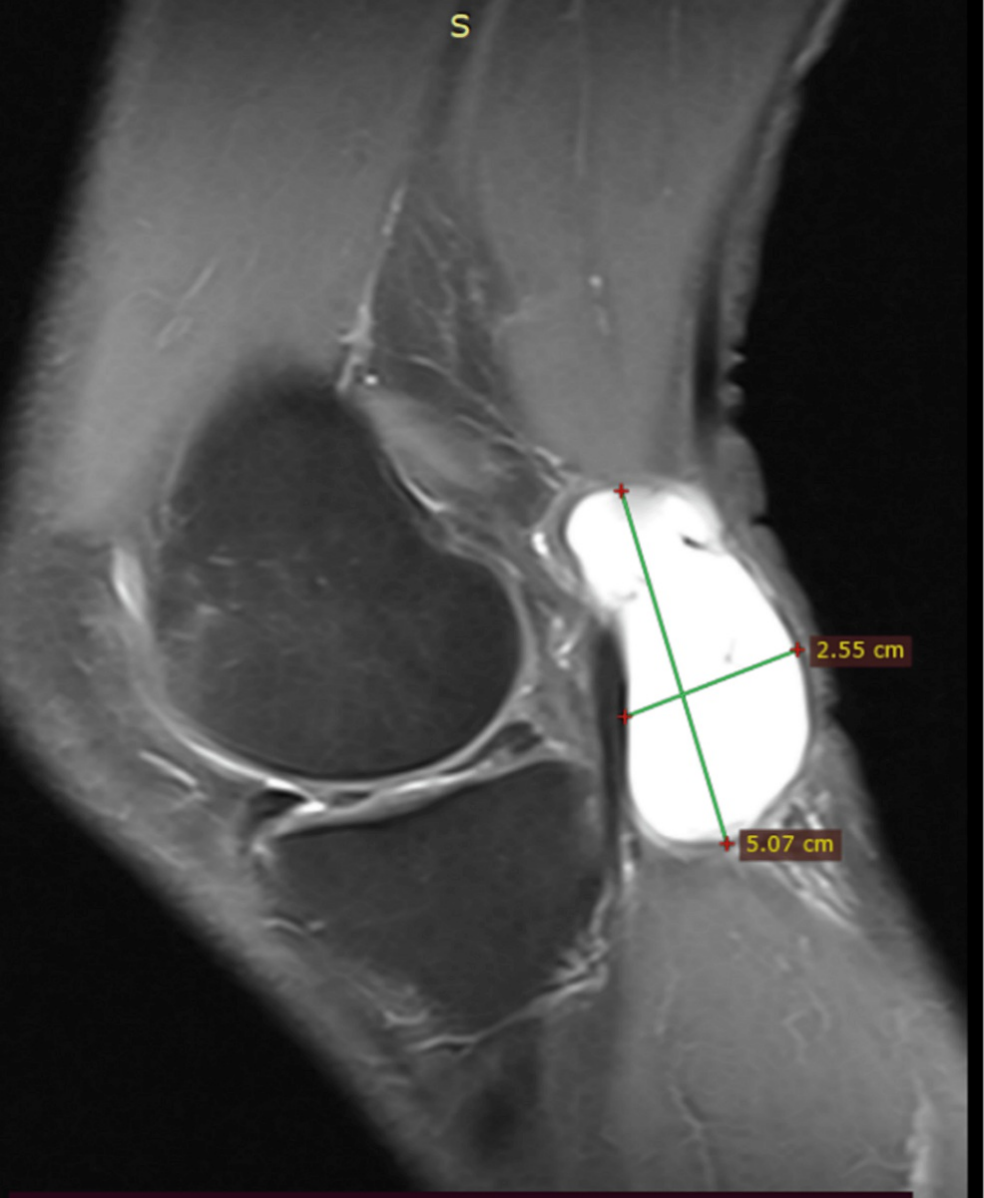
Sagittal T2-weighted image with increased signal of the cyst lying just lateral to the semimembranosus tendon in the popliteal fossa. Selected sample MRI studies demonstrate the size of the cyst in relation to adjacent anatomical structures.
